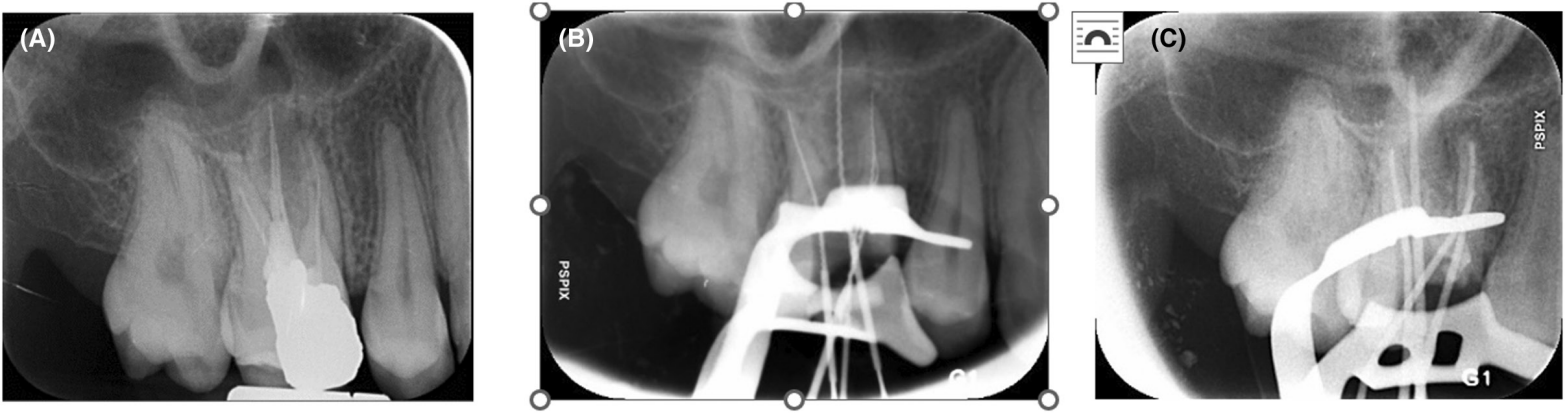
Periapical radiographs; diagnostic (A), initial file (B), cone fit (C).
